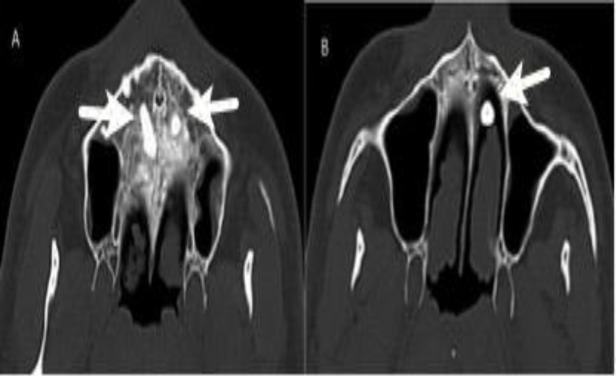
Axial section of paranasal CT
